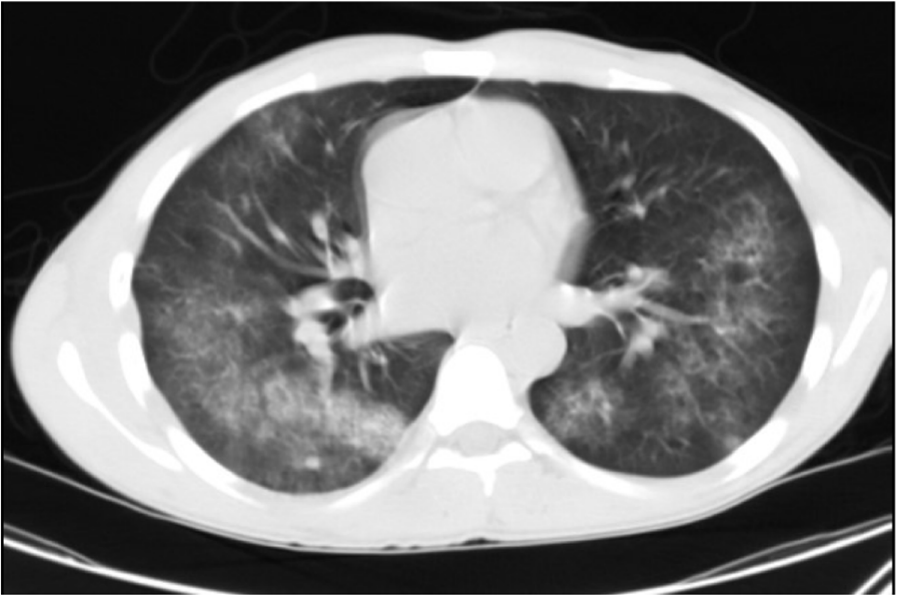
Pulmonary hemorrhage: thin section CT shows diffuse bilateral parahilar GGO without round configuration
